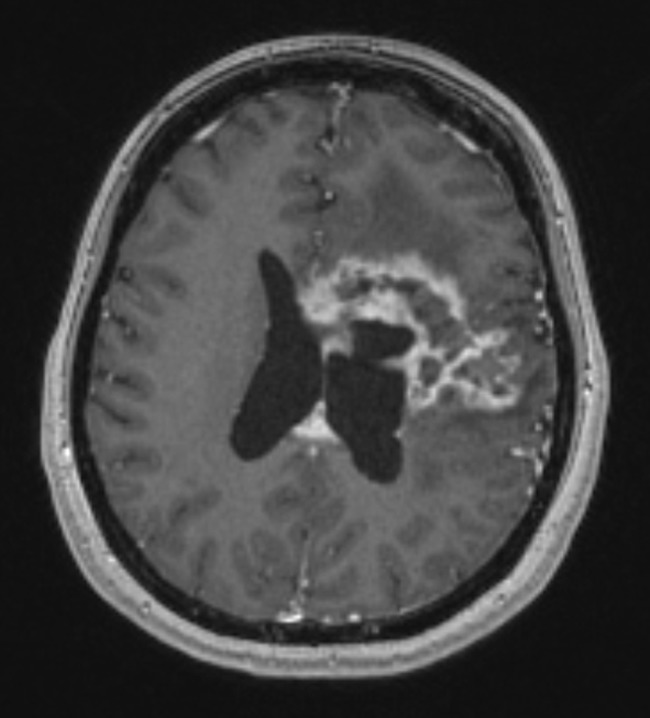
Example of a high-complexity lesion (5 points) on pre-operative contrast-enhanced T1-weighted MRI
